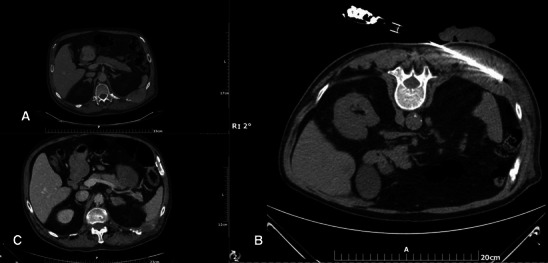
a Painful soft tissue mass infiltrating the left T10 posterior rib. b A microwave antenna is percutaneously inserted inside the mass. Due to the proximity to the skin a sterile glove filled with cold water is placed over the skin. c CT axial scan 3 months after the ablation session: Notice the significant size reduction of the mass along with new bone formation around the left T10 posterior rib (similar to heterotopic ossification)
